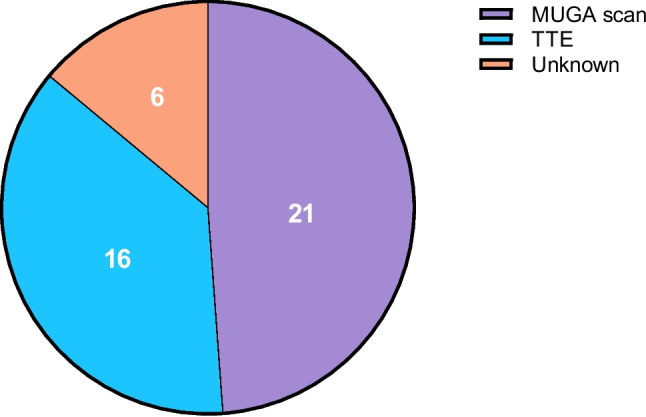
Diagnostic tools used for cardiac function evaluation during anthracycline therapy. Abbreviations: TTE = Trans Thoracic Echocardiography; MUGA = Multigated Acquisition
